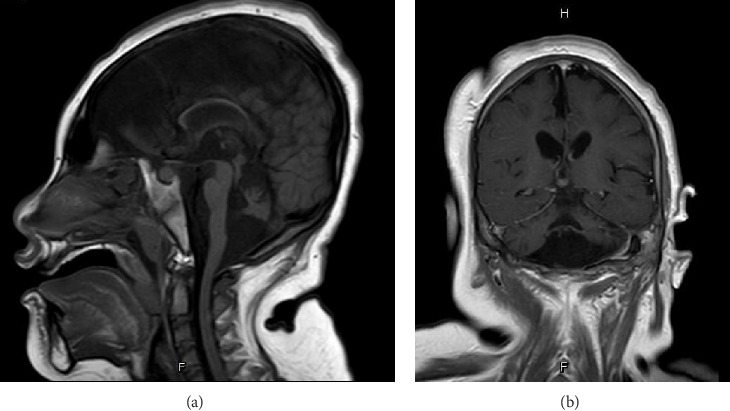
Brain MRI of the patient at 1 year of age. (a) Midsagittal T1-weighted image shows a thin corpus callosum, small pons, thinning of the medulla oblongata, and a hypoplastic cerebellar vermis. (b) Coronal T1-weighted image demonstrates a small cerebellar vermis and hypoplastic cerebellar hemispheres.
